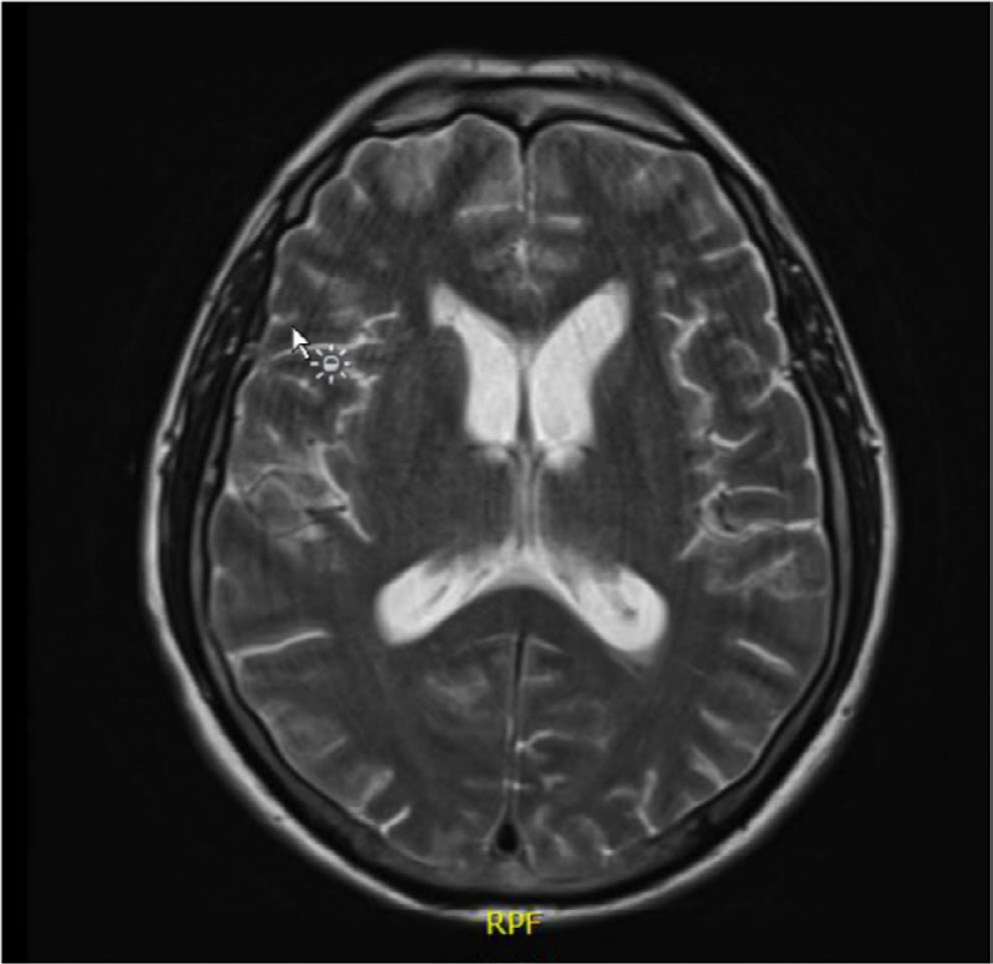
Transverse slice of repeat MRI Head (T2 weighted) on fourth presentation showing new hydrocephalus
